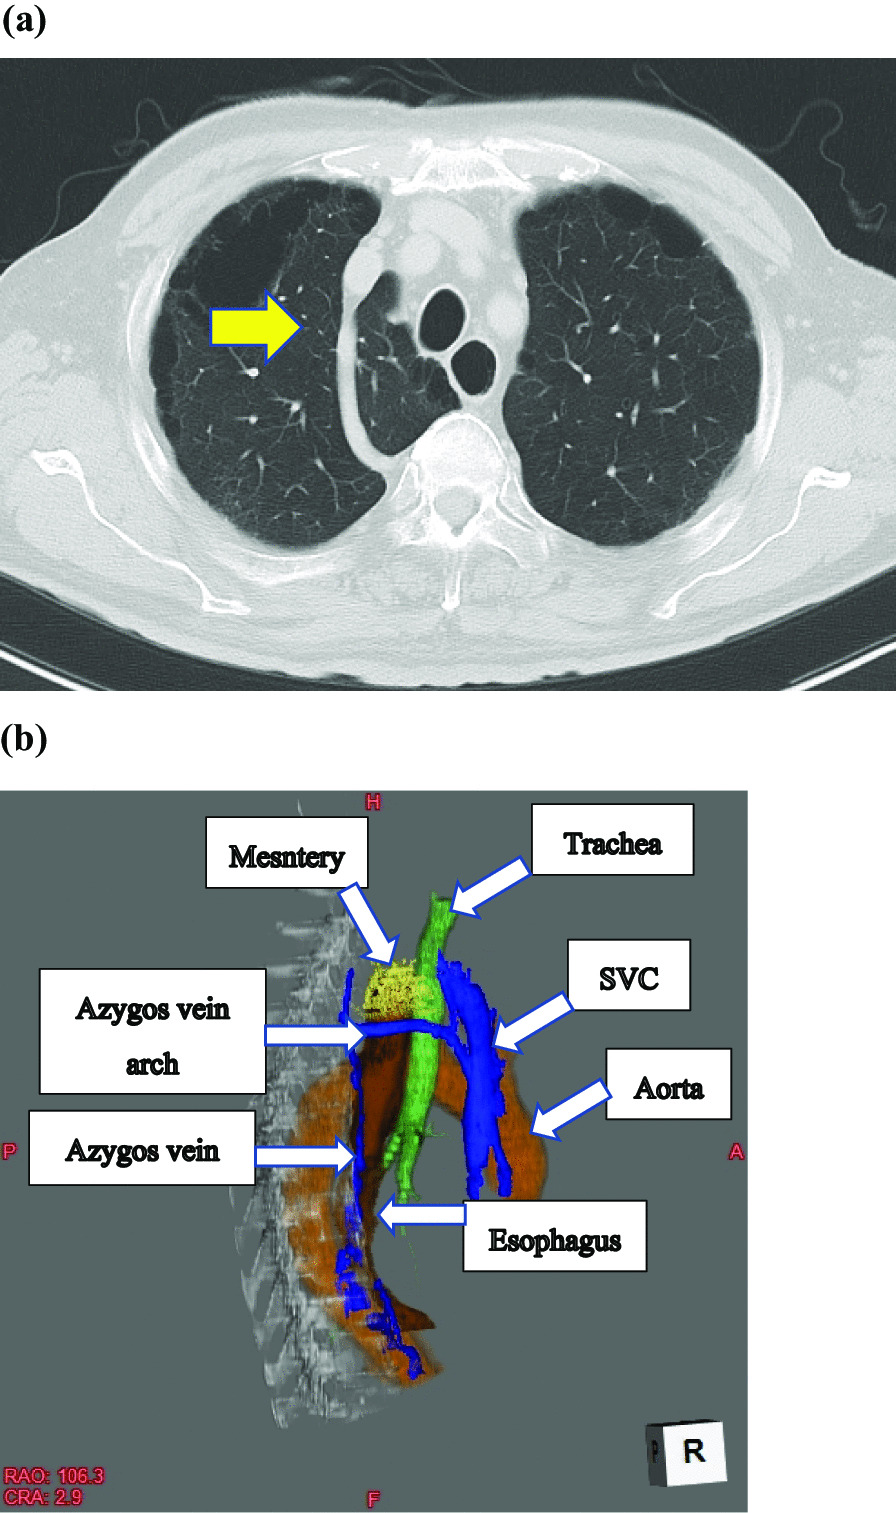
Azygos lobe. Axial image of CT (arrow) (a), and 3D-CT (b). SVC: superior vena cava
